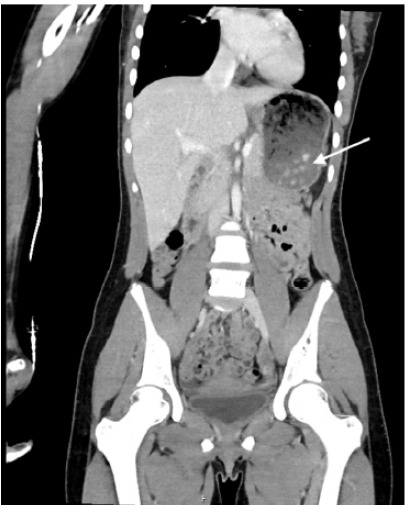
Coronal computed tomography with arrow demonstrating radiopaque foreign bodies within the stomach.
